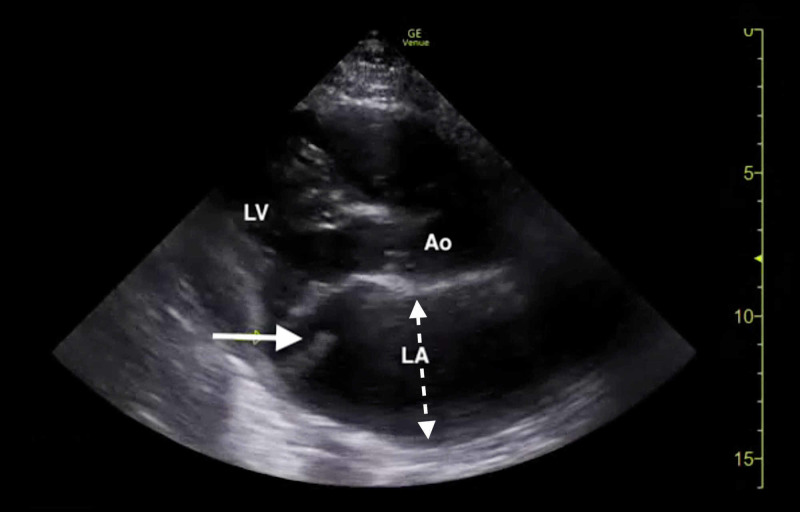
Bedside transthoracic echocardiogram (parasternal long-axis window) demonstrating a flail posterior mitral leaflet (solid arrow) and left atrial dilation (dotted arrow). Ao, aortic root; LA, left atrium; LV, left ventricle.
